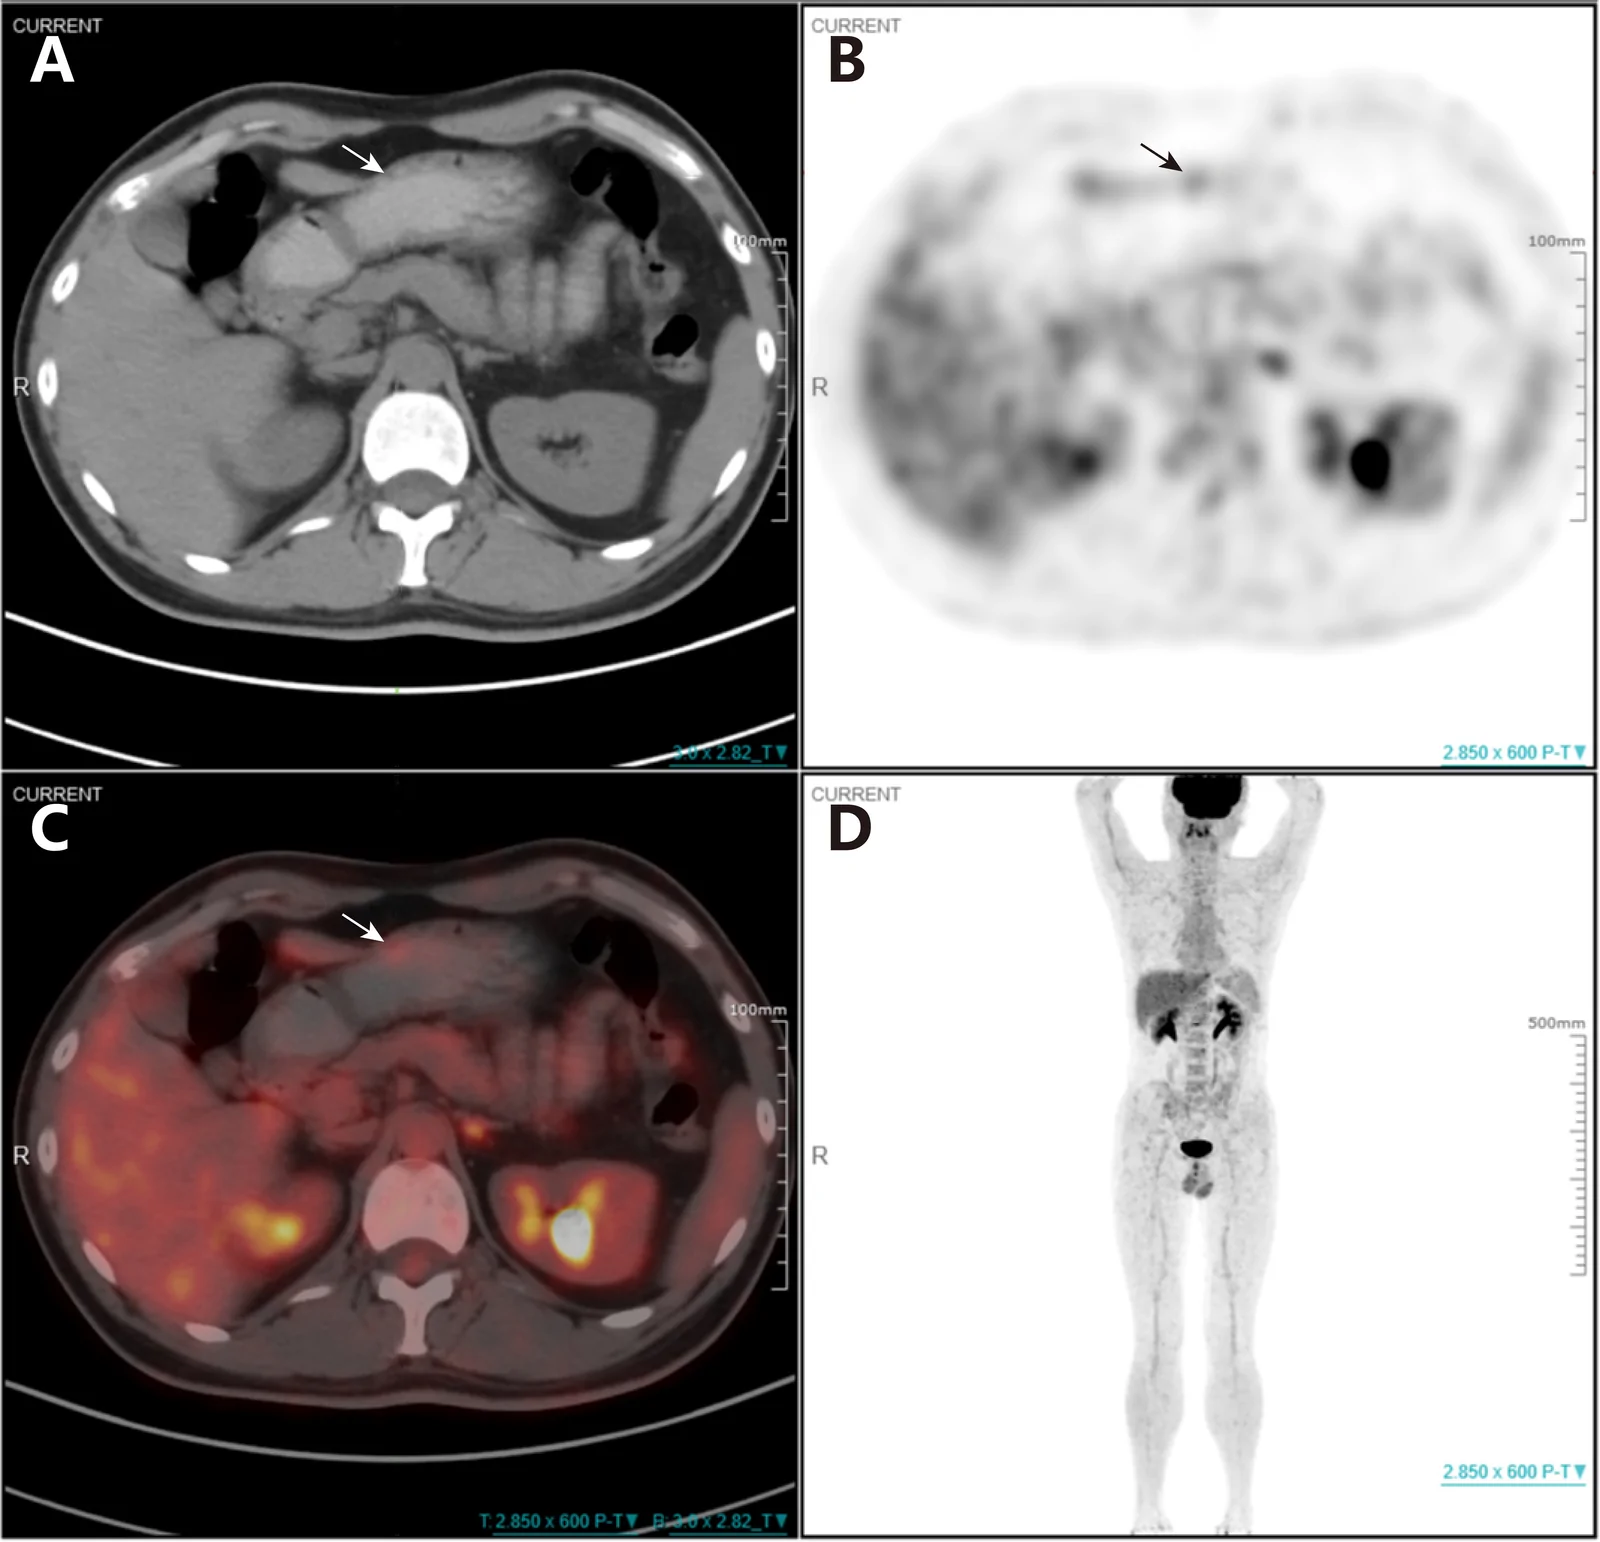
An abdominal CT scan is shown in Image (A), with the stomach indicated by a white arrow. Image (B) presents a PET scan, in which a gastric lesion with focally increased nodular FDG uptake is marked by a black arrow. The fused PET/CT image in Image (C) highlights increased metabolic activity in the anterior wall of the gastric antral greater curvature, with a maximum standardized uptake value (SUVmax) of approximately 2.7, as indicated by a white arrow. Finally, a maximum intensity projection (MIP) image from the whole-body PET scan is displayed in Image (D), which shows no evidence of lymphoma or other tumors.
